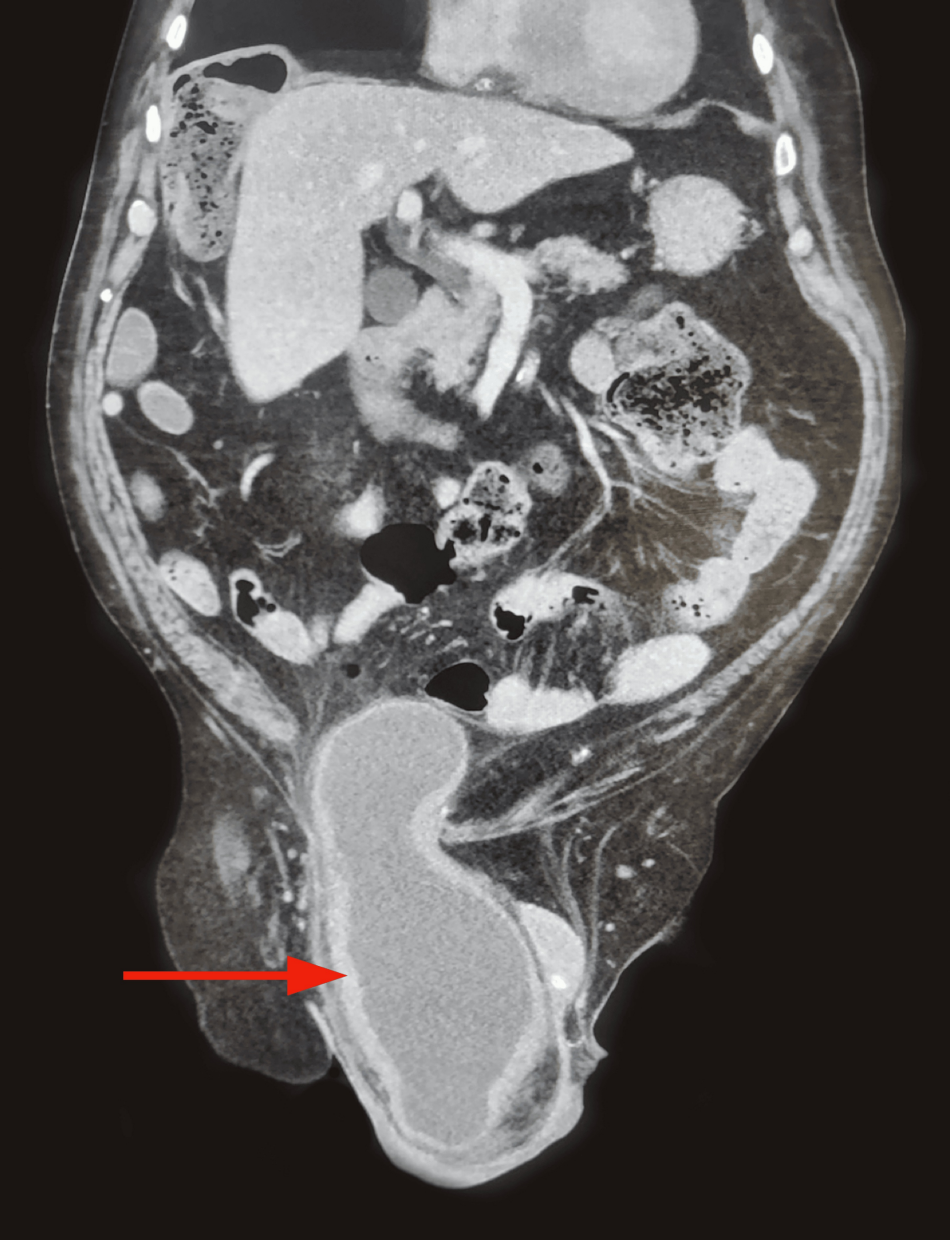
Sagittal section of an abdominopelvic CT scan demonstrating a bladder-containing inguinoscrotal hernia (red arrow).
